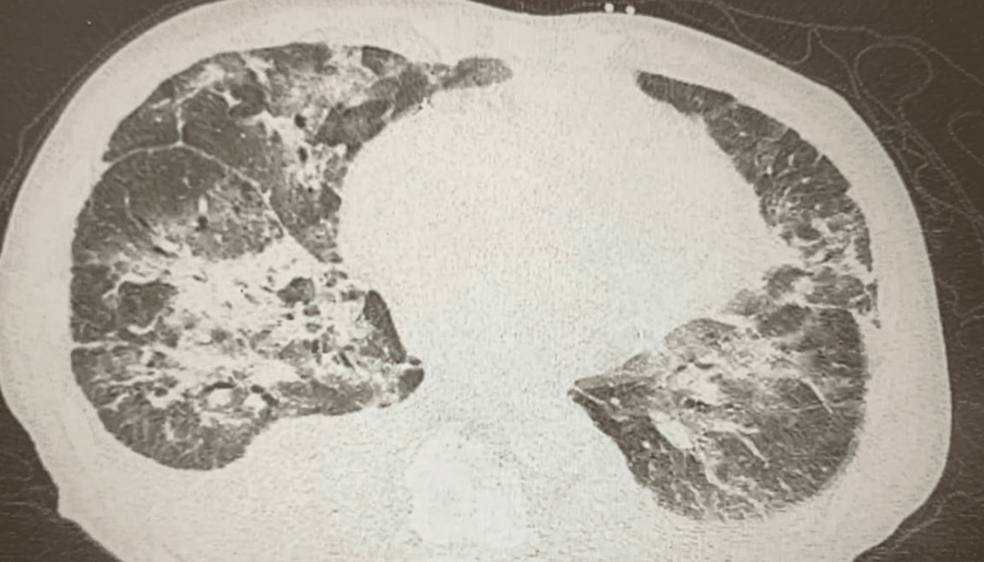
Chest CT imaging demonstrated multifocal, bilateral, hazy consolidations with increased interstitial markings and bilateral pleural effusions. CT: computed tomography
